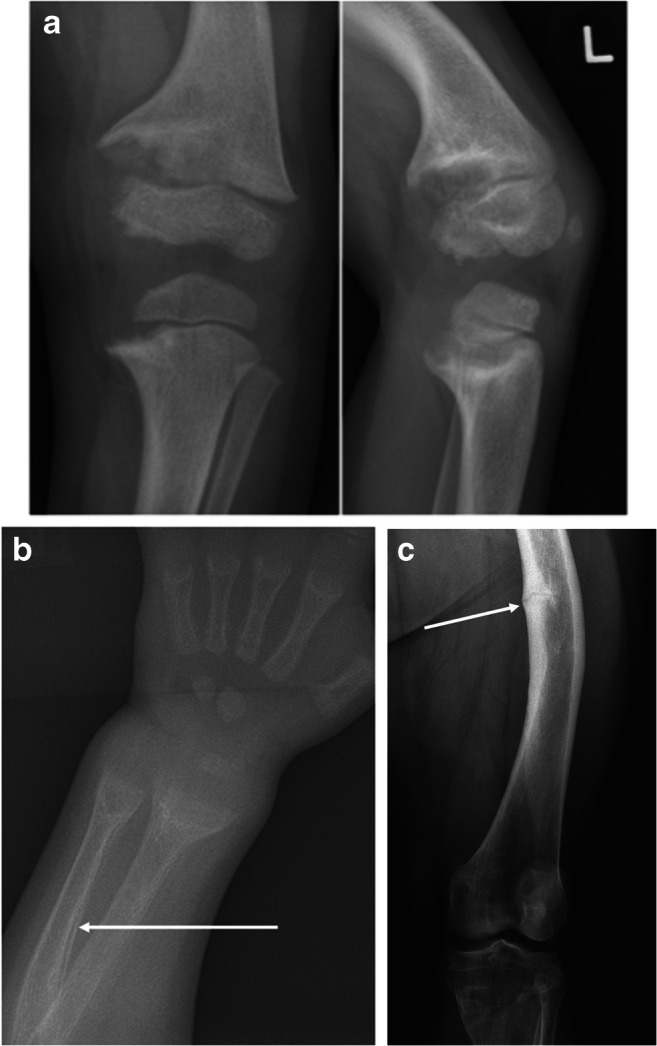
a Antero-posterior and lateral radiograph of the left knee in a 5-year-old boy with untreated XLH, widening, cupping, and fraying of metaphyses. Note the trabecular pattern at metaphyses and the asymmetry of the severity of the rachitic changes at the growth plate, with the medial side being more severely affected (wider and more frayed) than the lateral side. b Radiograph of the left wrist of a 2-year-old toddler with nutritional rickets secondary to severe vitamin D and dietary calcium deficiency. Note the florid and symmetrical widening, cupping, and fraying of metaphyses. The arrow points to periosteal reaction along diaphysis, due to severe secondary hyperparathyroidism. c Radiograph of the left femur from a 23-year-old man with XLH showing a medial diaphyseal pseudofracture or “Looser zone” (arrow)
